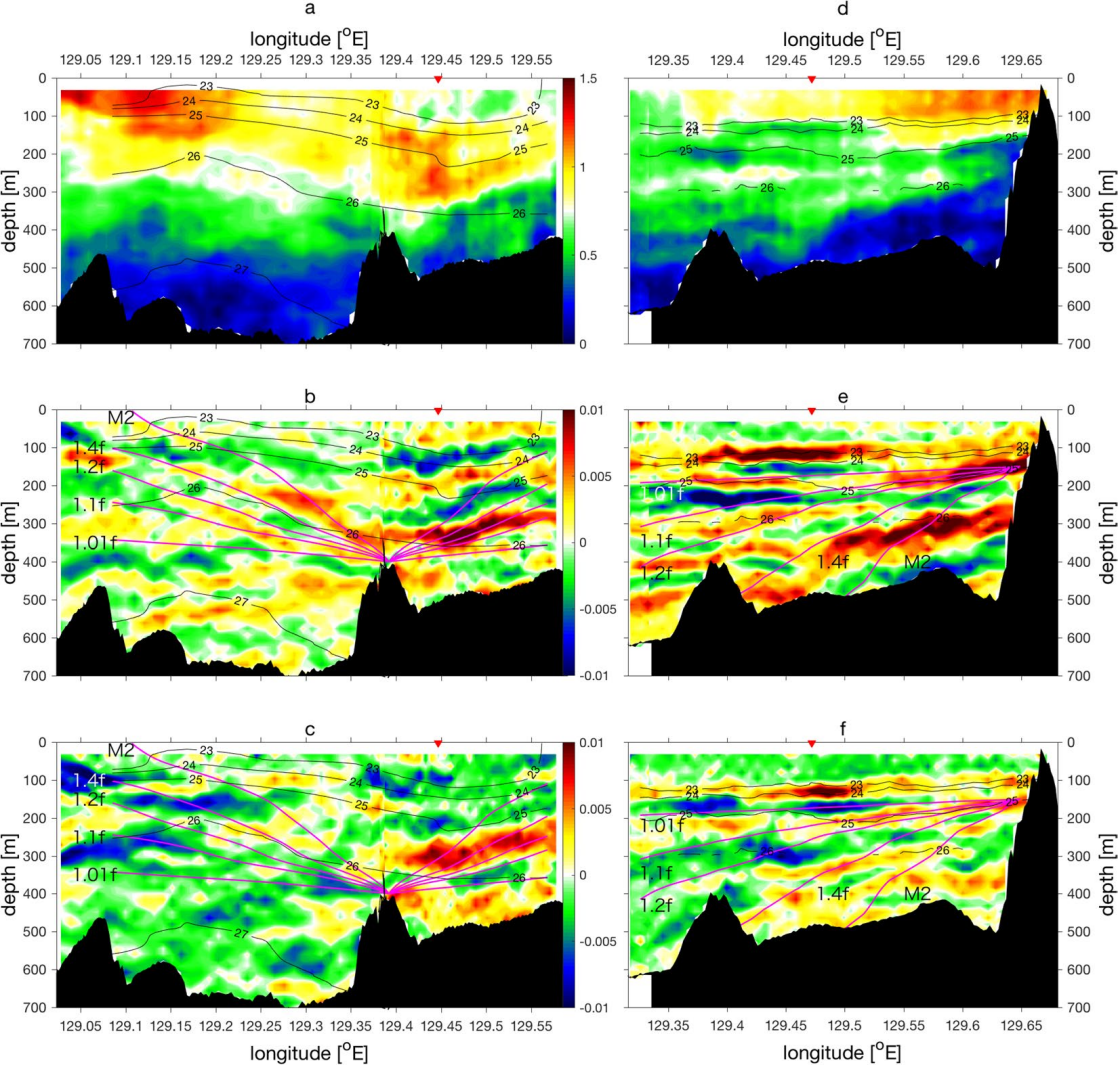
Vertical sections of the shipboard Acoustic Doppler Current Profiler velocity measurements. (a–c) are for Leg A (blue in Fig. 1b) and (d–f) are for Leg B (red in Fig. 1b). Horizontal absolute current velocity (m s^−1^) are shown in (a,d). Back-rotated shear [s^−1^] is shown for zonal shear *u*
